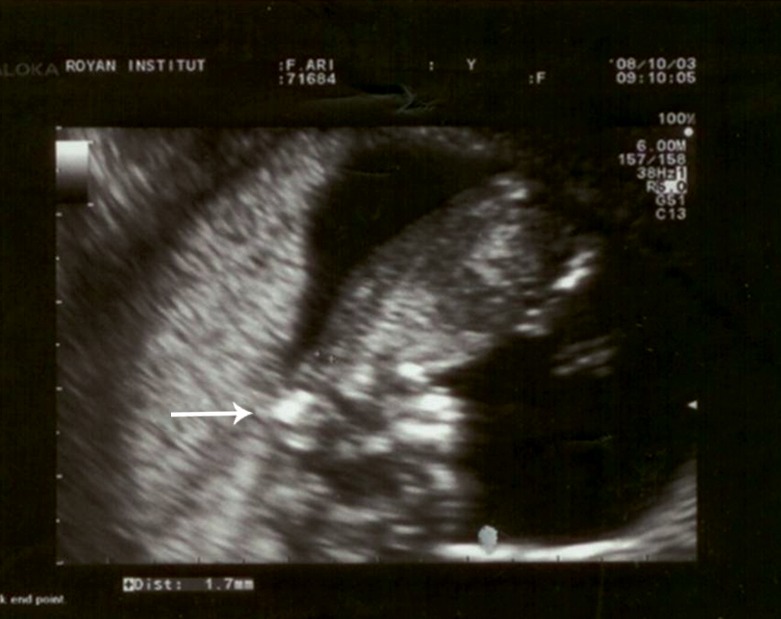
First trimester sonography in a 26-year old pregnant
